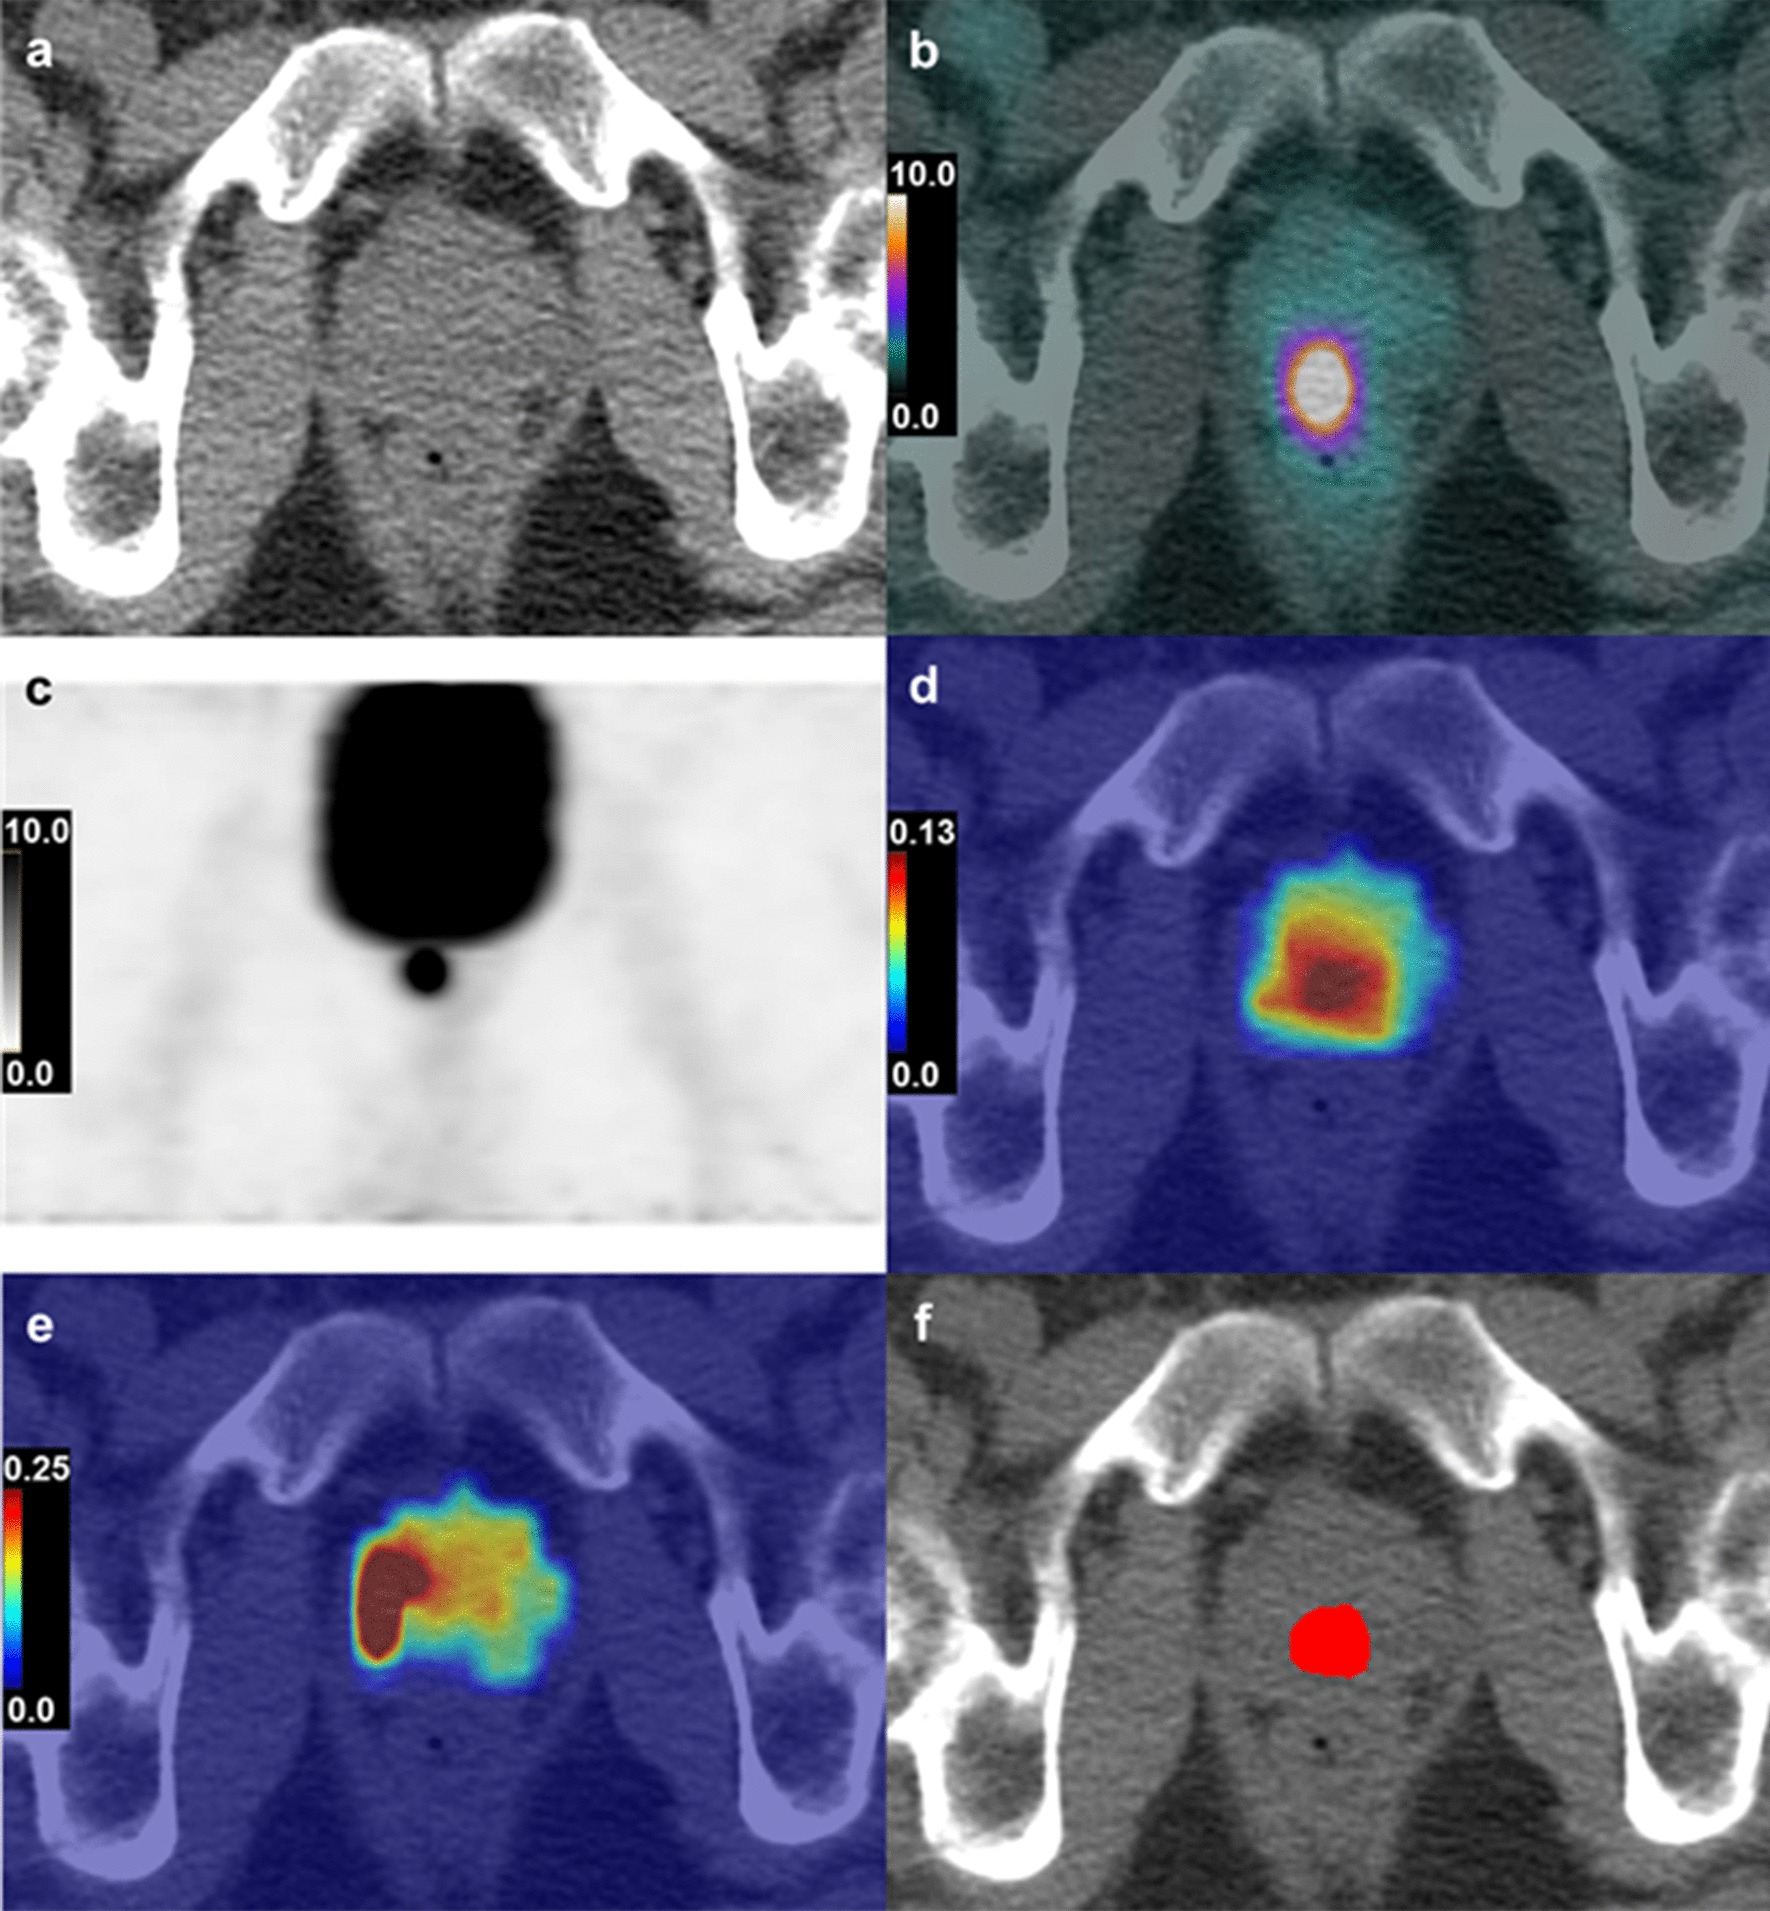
Axial CT image (a), axial [^18^F]DCFPyL SUV map (range 0–10.0 g/mL) superimposed on CT (b), coronal [^18^F]DCFPyL PET (range 0–10.0 g/mL) (c), K_i_ parametric map (d), *k*_4_ parametric map (e) and localized tumour in red using the classifier from logistic regression (f), in a 64-year-old man with PCa (Gleason score 7 (3 + 4)). Pre-op PSA was 8.17 ng/mL. Mean SUV of the tumour ROI was 13.4 g/mL. CT, computed tomography; DCFPyL, 2-(3-{1-carboxy-5-[(6-[^18^F]fluoro-pyridine-3-carbonyl)-amino]-pentyl}-ureido)-pentanedioic acid; SUV, standardized uptake value; PET, positron emission tomography; *K*_i_, net uptake rate constant from plasma; *k*_4_, dissociation rate constant; PCa, prostate cancer; PSA, prostate-specific antigen; ROI, region of interest
